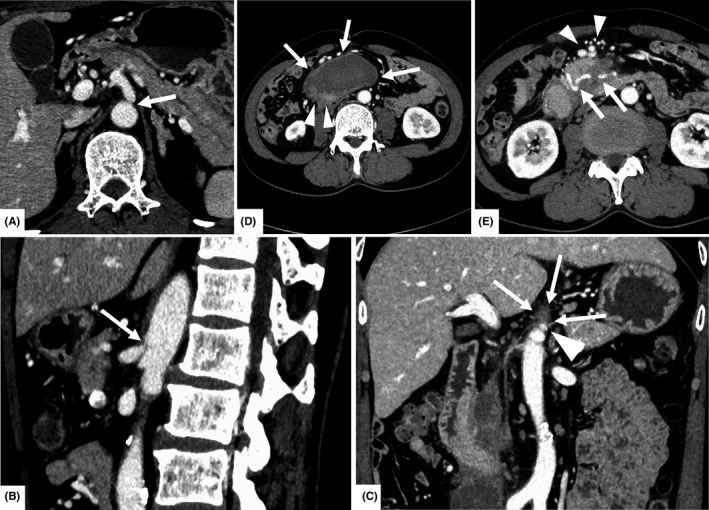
Abdominal computed tomography with contrast enhancement. A, Cross‐section image shows severe stenosis at the origin of the celiac artery (arrow). B, Sagittal section image shows severe stenosis at the origin of the celiac artery more clearly (arrow). C, Coronal section images show severe stenosis at the origin of the celiac artery (arrowhead) caused by the midline arcuate ligament (arrows). D, A 5‐ × 6‐cm low‐density mass (arrows) encloses the third portion of the duodenum (arrowheads) without definite extravasation of contrast material. E, Dilatation of the branches of the pancreaticoduodenal artery (arrows) and collateral circulation (arrowheads) is revealed.
